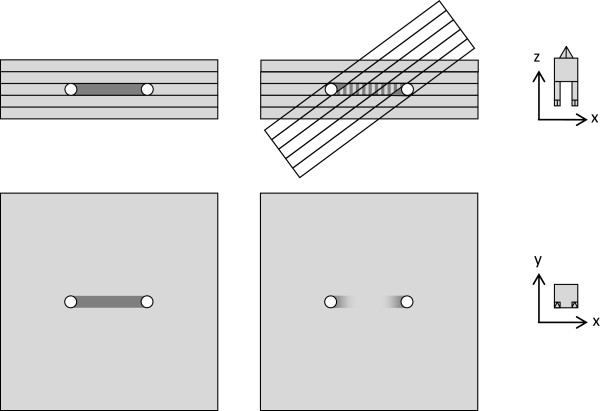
An additional tilted CT provides complementary information. Left top (coronal plane): a reconstructed CT consists of a stack of slices (light grey bars), and one of the slices contains two opaque bodies (white circles). Left bottom (transverse plane): this slice containing the opaque bodies is prone to feature a streaking artifact between them (dark grey area). Right top (coronal plane): An additional CT which is tilted against the first one offers complementary information from a different angle, in particular, the dark grey area is partially covered by slices which do not contain any opaque bodies. Right bottom (transverse plane): as a result, when averaging over two or more CTs with complementary information, streaking artifacts are reduced.
